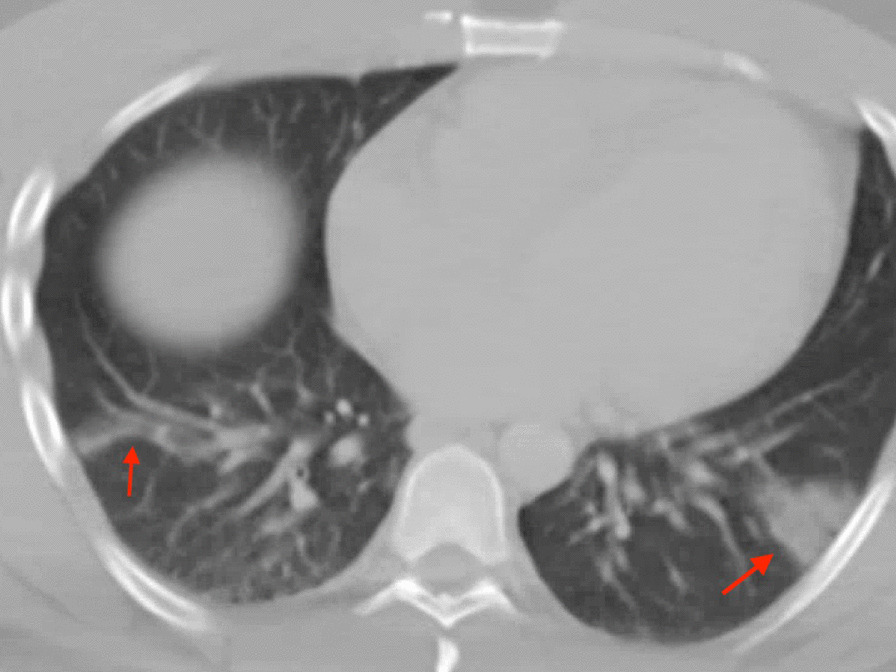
Case 6. Figure 1. Axial chest computed tomography (CT). Bilateral pulmonary consolidations (red arrows) present
